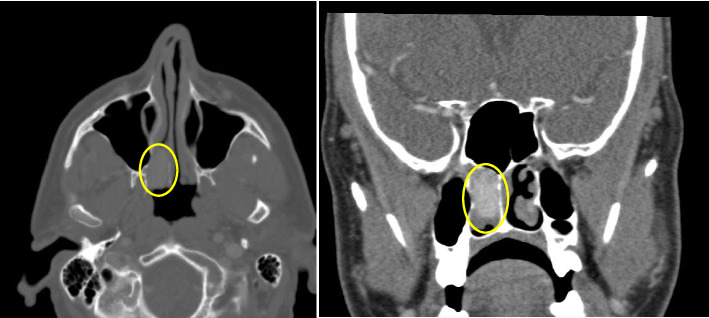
CT sinus of the sinonasal mass on presentation. The lesion is outlined in each image with a yellow circle.
